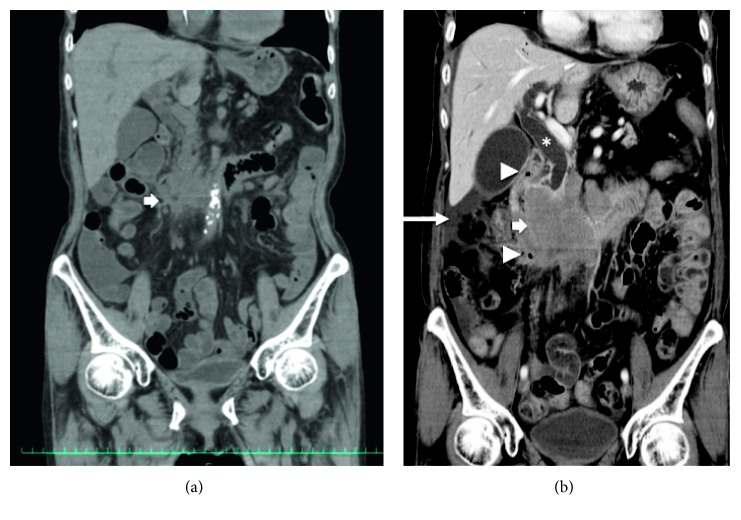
Contrast abdominal CT (coronal) image upon admission. The scan shows (a) prominent thickening of the duodenal wall from the second to third portion (arrow), and (b) perforation 17 days after gastrojejunostomy revealing an enlarged duodenal mass (arrow), free air (arrow heads), ascites (long arrow), and expansion of the common bile duct/intrahepatic bile duct (^∗^) due to tumor-related stenosis.
